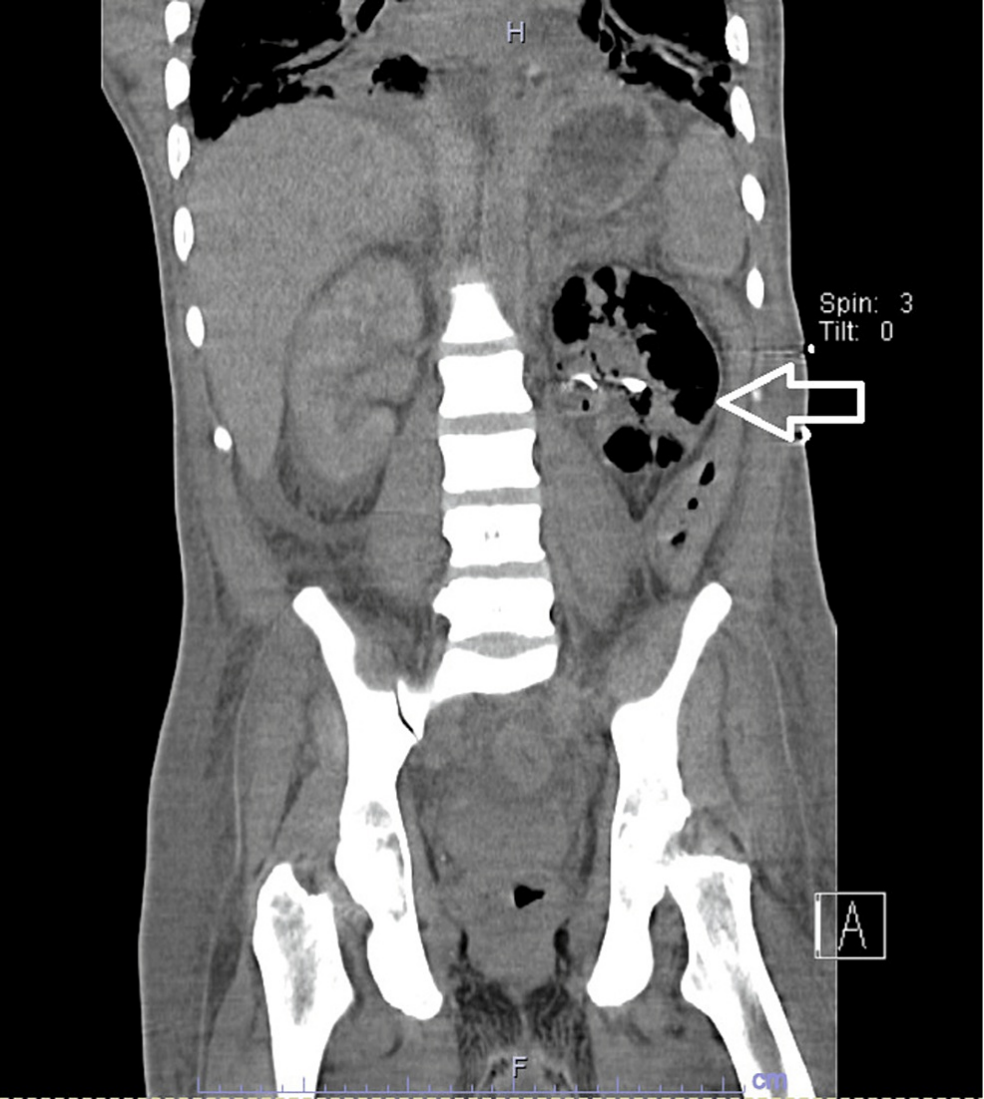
CT Scan of the Abdomen and Pelvis, 48 Hours Post Left Nephrostomy Tube Placement, Showing Progression of EPN in the Left Kidney (Arrow)
